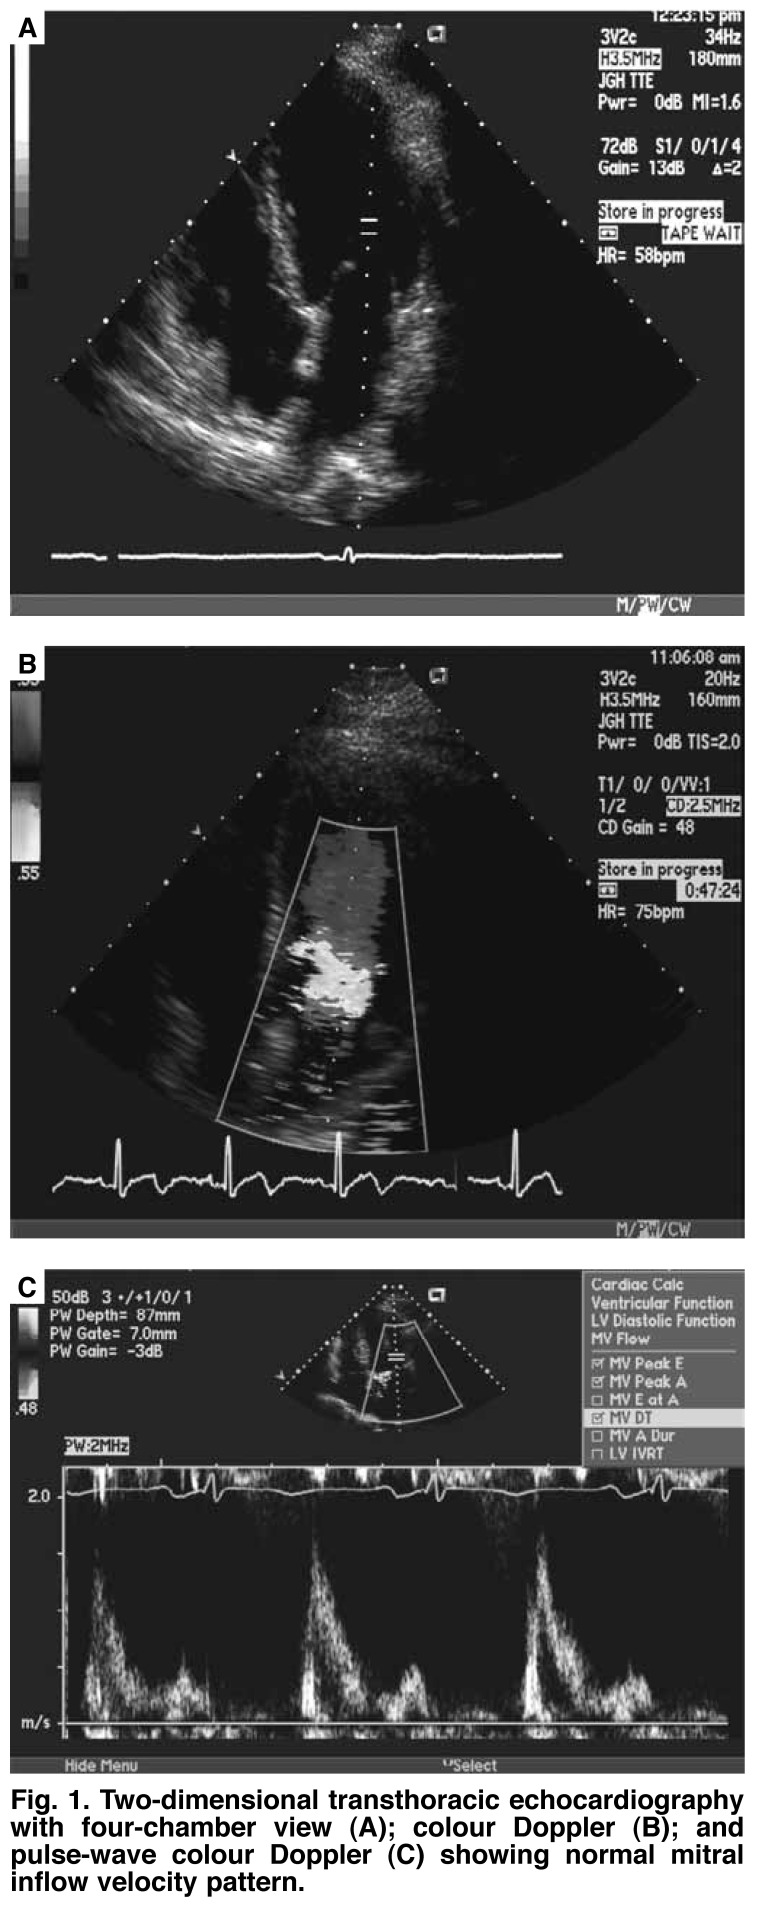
Two-dimensional transthoracic echocardiography with four-chamber view (A); colour Doppler (B); and pulse-wave colour Doppler (C) showing normal mitral inflow velocity pattern.
